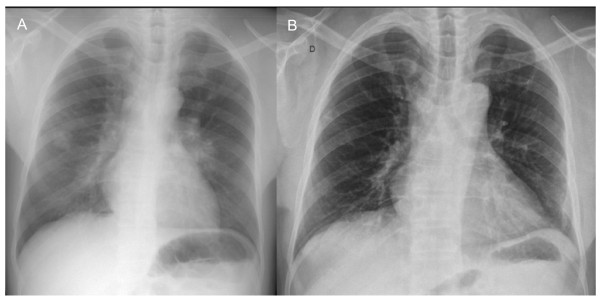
Chest radiograph of case 2. A: At presentation, alveolar consolidations predominant to right lung and parahilar left area. B: Major improvement after 2 years of treatment.
